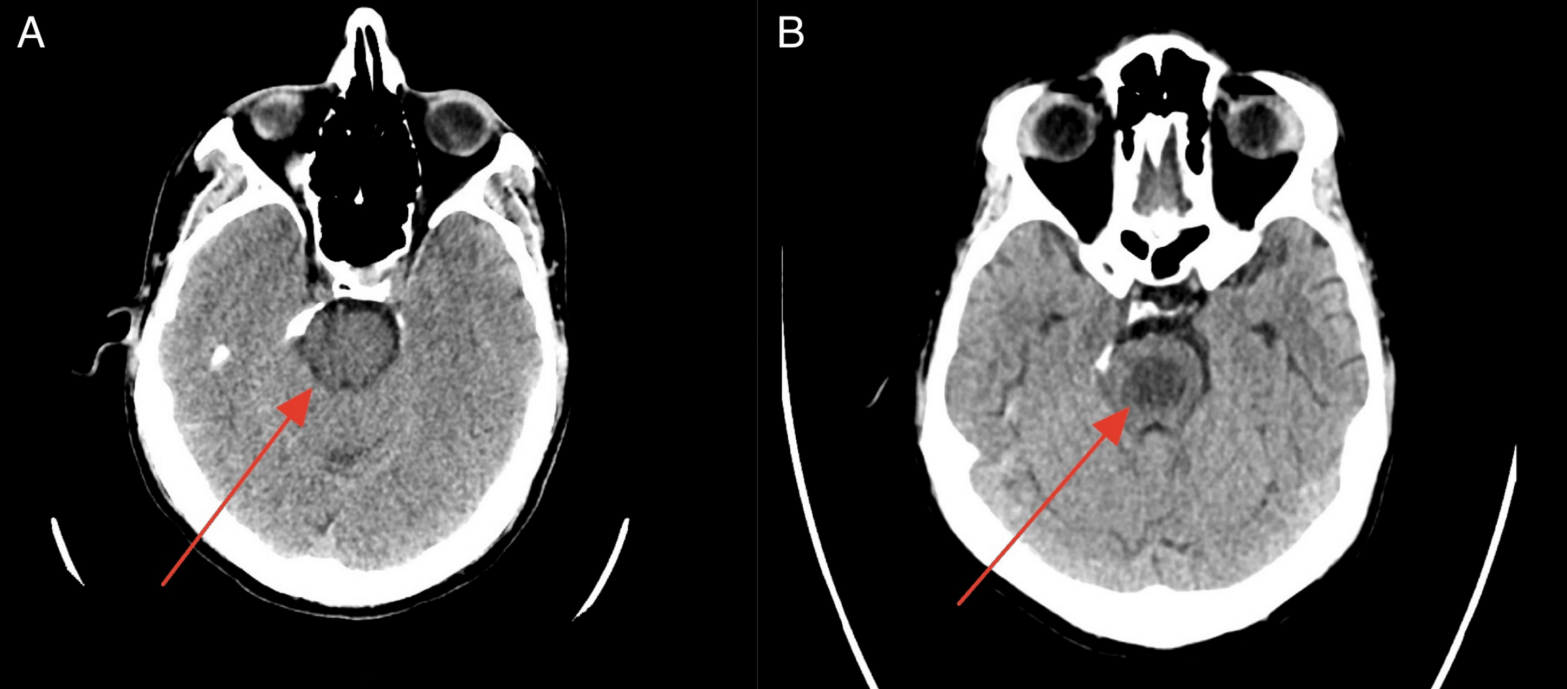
A normal CT scan performed upon the patient’s admission showed no detectable changes in the brain (A), while a follow-up CT scan after sodium correction revealed rounded hypodensities in the pons (B).
